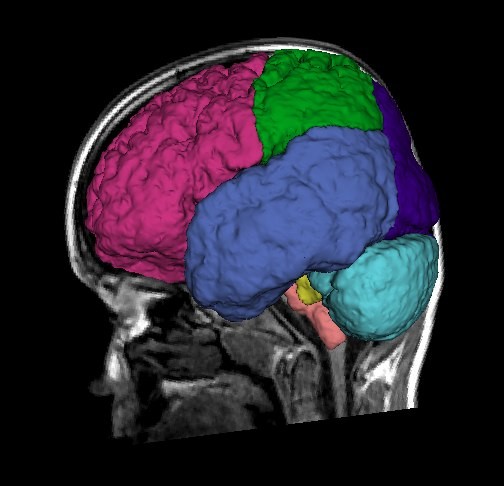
3D rendering of parcellation of a 9-year-old child’s brain from MRI. The surface model is depicted on top of a mid-sagittal slice from the T1-weighted MRI. Comparison of regional tissue volumes across subjects can be employed to indicate localized structural differences.
